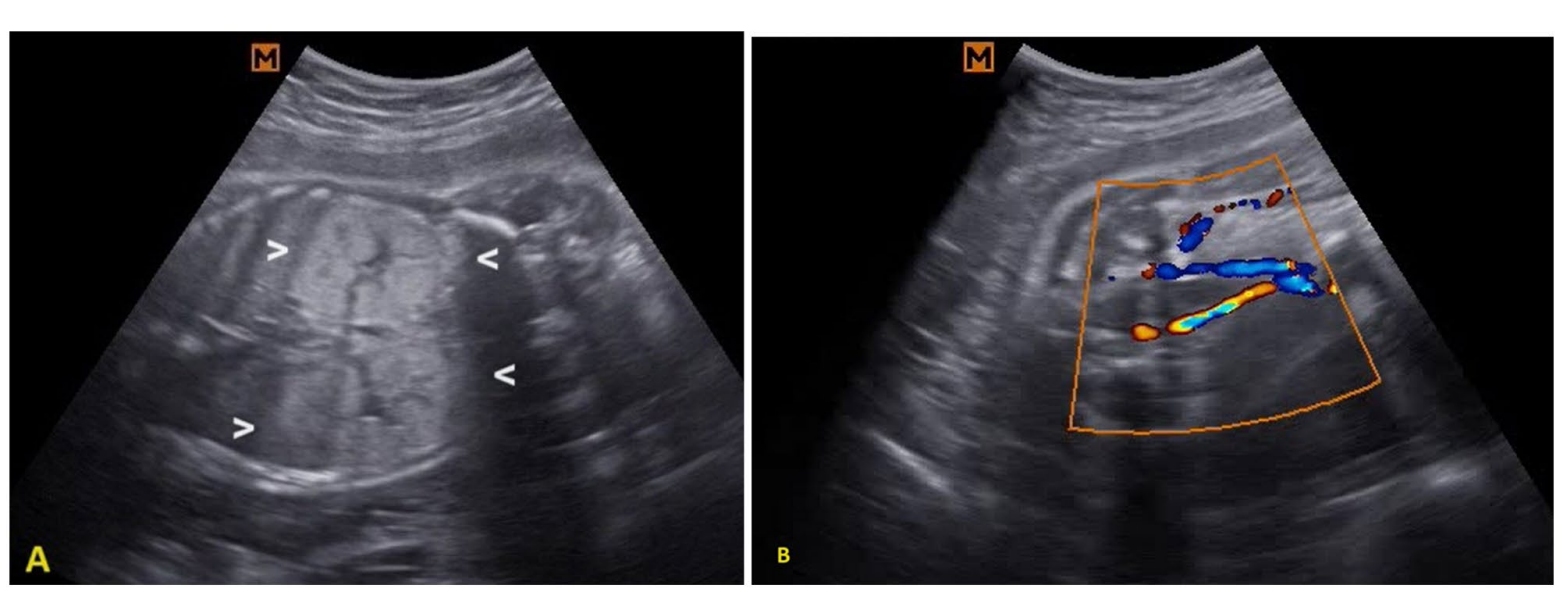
Prenatal 2D grey scale ultrasound. A Coronal view of the bilateral enlarged echogenic fetal kidneys with loss of corticomedullary differentiation; B Color Doppler ultrasound of umbilical arteries lining the small urinary bladder
